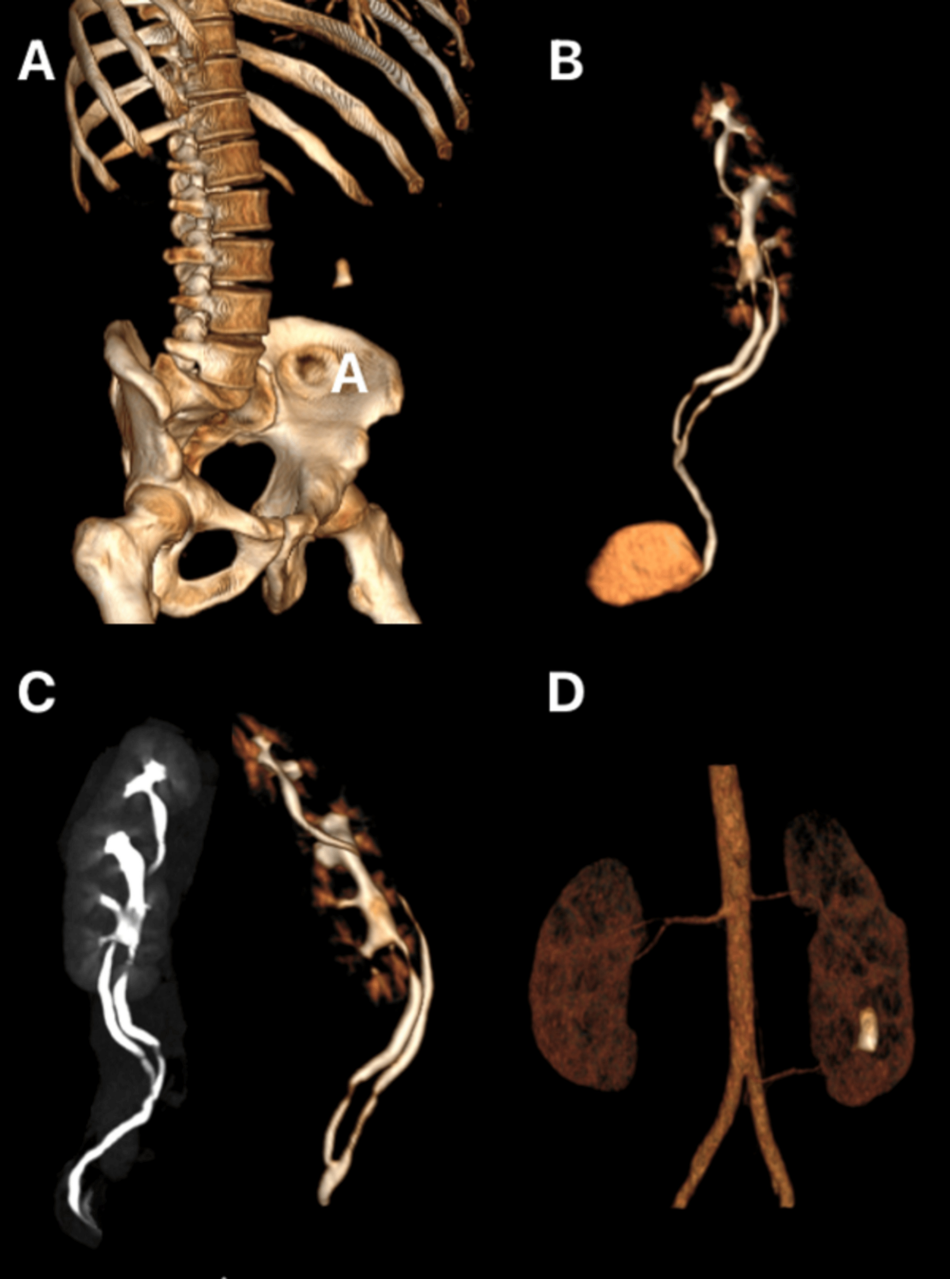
Angiotomography CT (A) 3D reconstruction showing just a large calculus. (B) 3D reconstruction of the urinary system's collecting system featuring the two separate renal pelvises and two ureters that unite (incomplete). (C) 3D reconstruction of the left supernumerary fused kidney. (D) Angiotomography CT: angiography reconstruction showing the vascular supply of the three kidneys (right, left, and ipsilateral supernumerary) with one artery each. The supernumerary (superior) kidney artery is identified as a direct branch of the aorta, and the native (inferior) kidney artery is a branch of the left common iliac artery.
